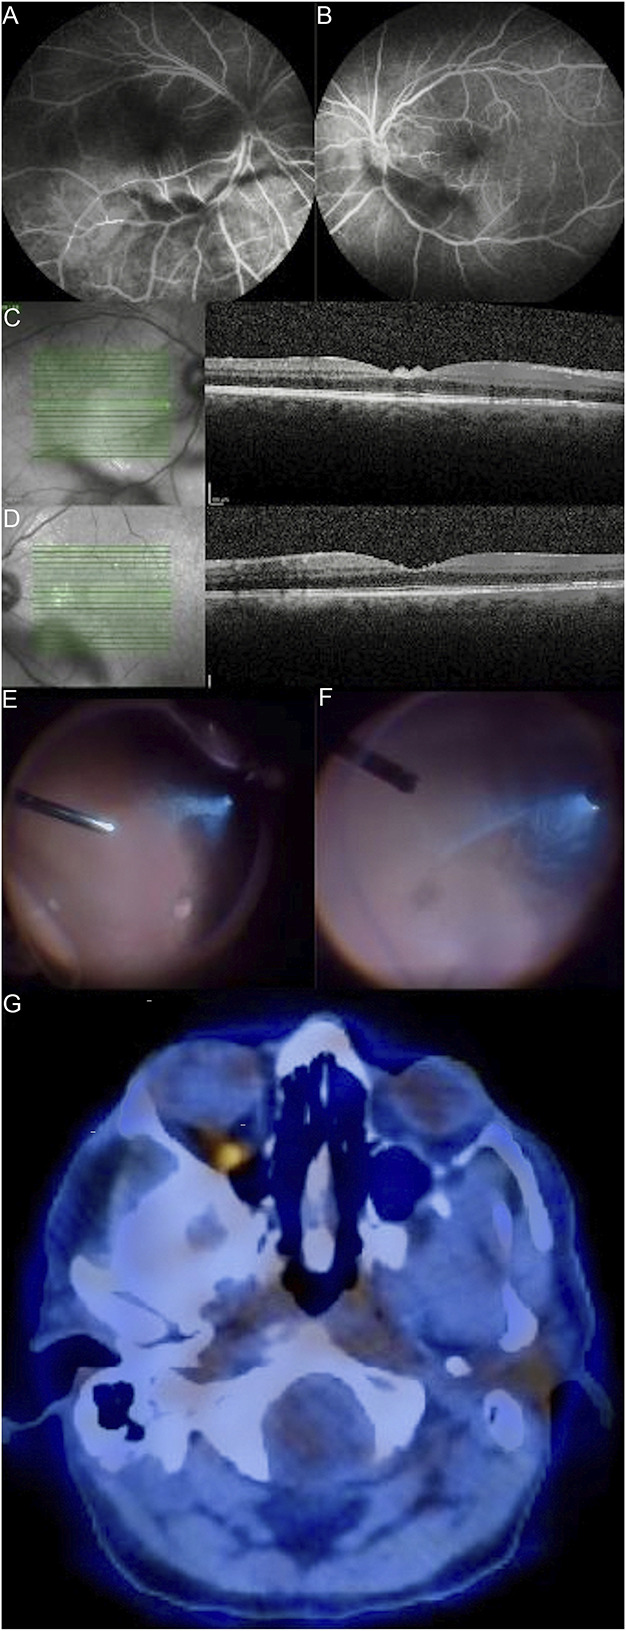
Fluorescein angiography (A and B, respectively) and optical coherence tomography (C and D, respectively) images of the right and left eyes presenting moderate vitritis, with no signs of retinal or choroidal involvement. Pars plana vitrectomy images from each eye (fourth row) showing the appearance of intermediate/large B-cell lymphoma cells in the vitreous cavity, corresponding to homogeneous, large cell sheets that resemble an “aurora borealis” pattern. Positron emission tomography scan (G) revealing asymmetry in the uptake of FDG-F18 in both ocular globes, with increased uptake in the right eye.
